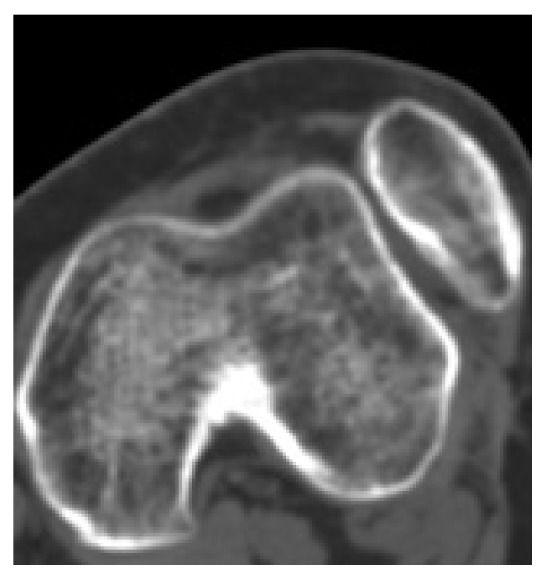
Pre-op knee CT demonstrating patella dislocation.
